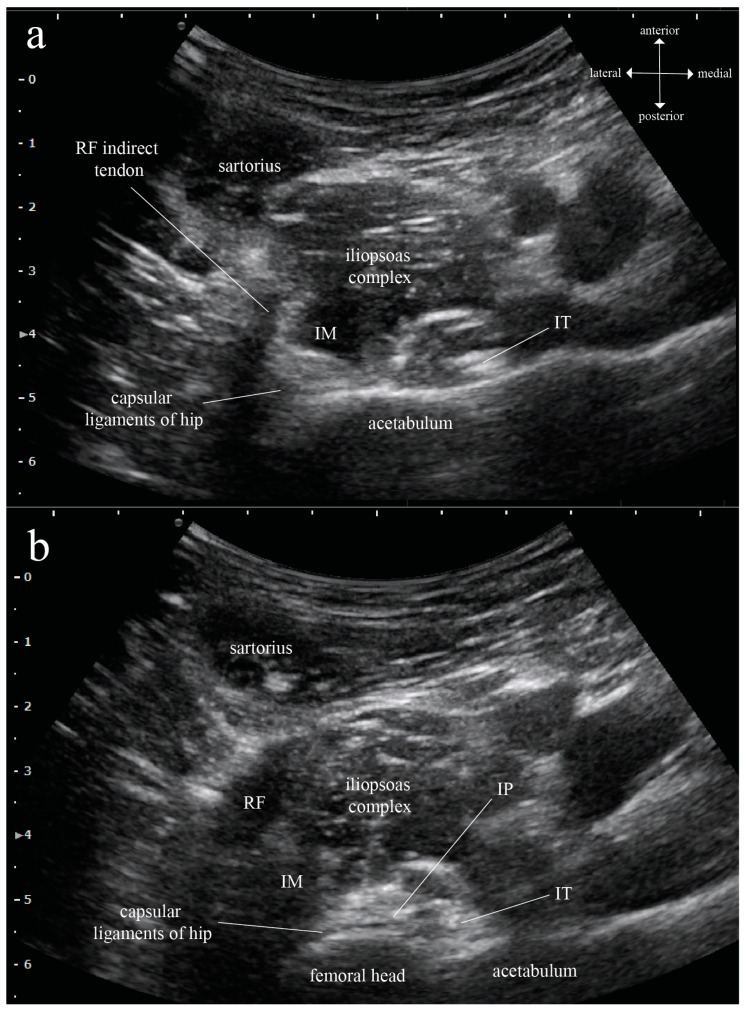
Ultrasound transverse scan of the human right hip with a curvilinear probe: (a) At the level corresponding to Figure 1a, the rectus femoris (RF) indirect tendon is an easily identifiable, elongated hypoechoic shadow lateral to the iliopsoas complex that can be used as a startup sonographic landmark to identify the iliopsoas plane (IP) at the level of iliopsoas plane block (IPB) and anterior hip pericapsular neurolysis. Under ultrasound, the iliopsoas complex and the capsular ligaments of hip are both hypoechoic, while iliopsoas tendon (IT) is a hyperechoic ovoid structure situated at the medial corner of iliopsoas complex. (b) At the level corresponding to Figure 1b, in between the iliopsoas complex and capsular ligaments of hip, the IP appears as a fuzzy, hyperechoic layer. The IM medial border is, in general, very difficult to identify sonographically, making the lateral margin of IP obscure and hard to define on ultrasound.
